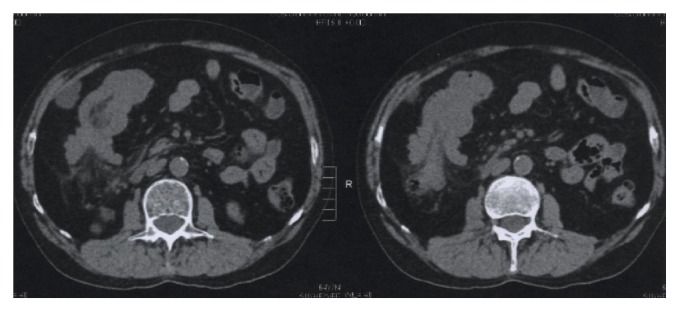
Abdominal CT (11/2012), intussusception of the right colon, proximal to the transverse colon and hepatic flexure.
